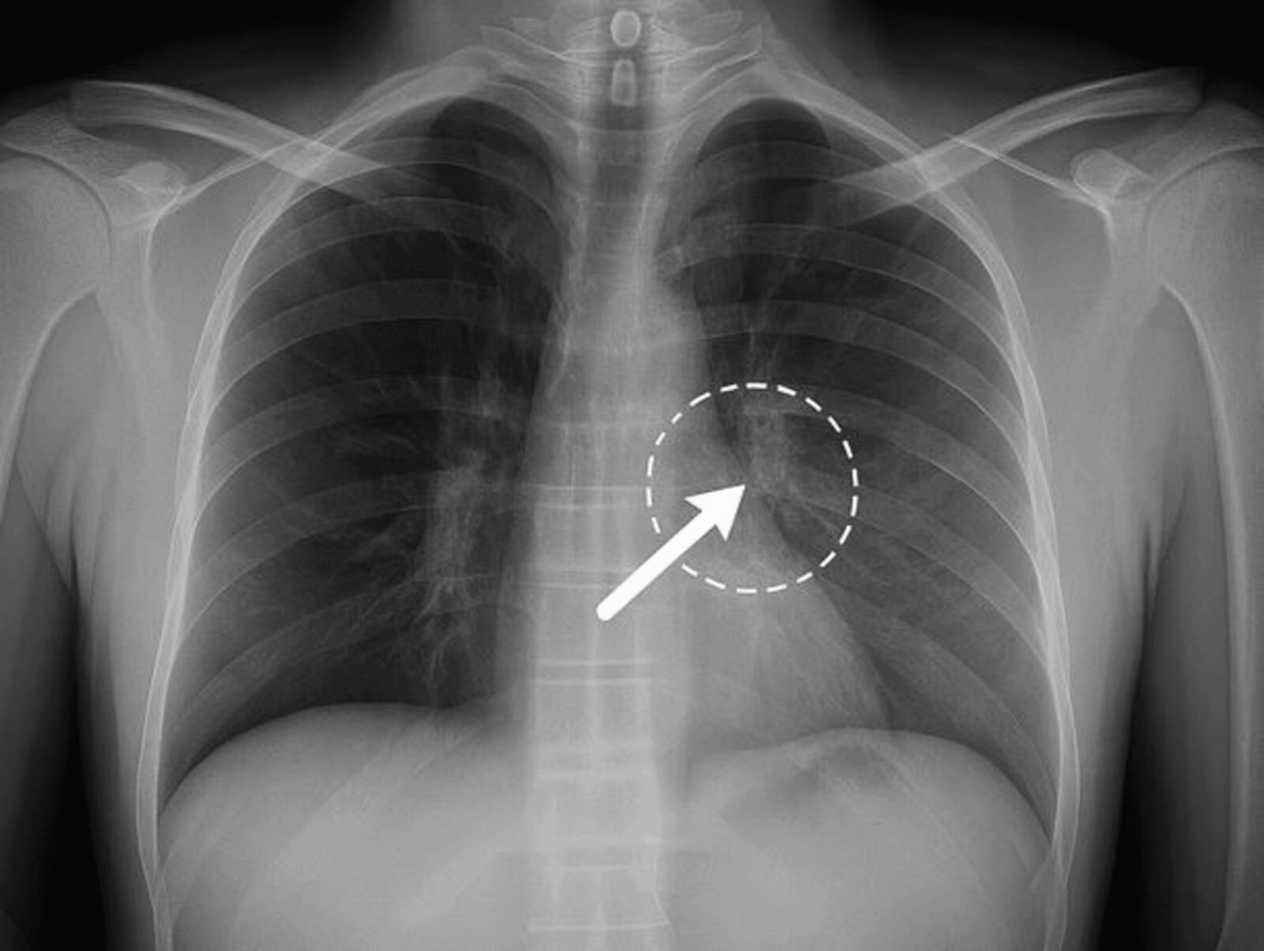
Chest radiograph demonstrating a subtle interstitial infiltrate in the left upper lobe (LUL), consistent with mild lower respiratory tract involvement. The arrow and dashed circle indicate a subtle interstitial infiltrate in the LUL. There was no evidence of consolidation, pleural effusion, or significant hyperinflation.
